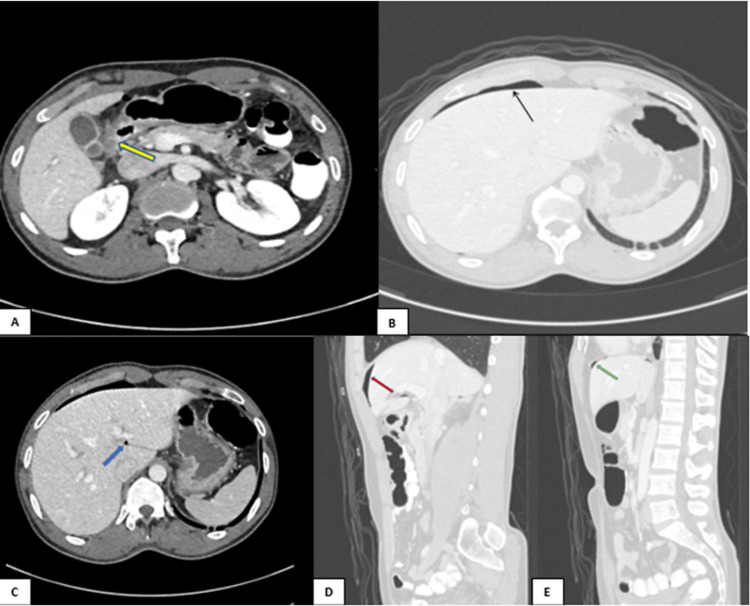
CT abdomen with peroral and IV contrast (A-C) axial and (D, E) sagittal reformatted images showing mural thickening, irregularity, and abnormal wall enhancement involving the pyloric region and the first part of the duodenum (yellow arrow) with a trace of pericholecystic fluid and fat stranding surrounding the antrum and proximal duodenum. There is intraabdominal free air along the porta hepatis (blue arrow), anteriorly in the upper abdomen (red arrow), and in the subdiaphragmatic region (green arrow).
